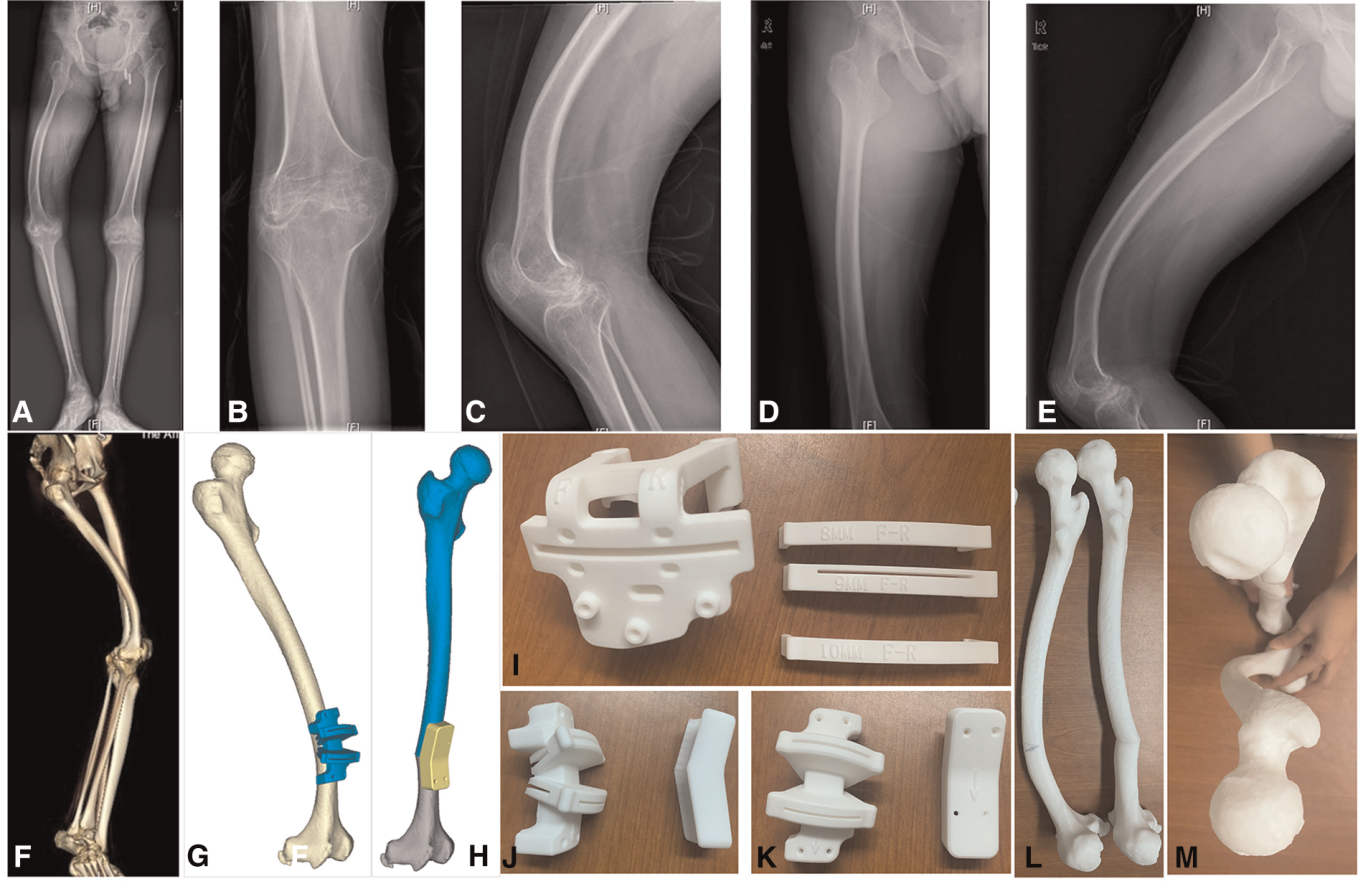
(A) Full-length x-rays of both lower limbs (weight-bearing); (B–E) anteroposterior and lateral x-rays of the knee joints and femur. The sagittal deformity angle of the femur was 30° (anterior); (F) full-length computer tomography film of right lower limb; (G,H) computer model of distal femoral osteotomy; (I) distal femur osteotomy guide plate in TKA; (J, K) osteotomy and reduction guide plate for extra-articular deformities; (L,M) models of femur in lateral and overhead positions after the osteotomy.
